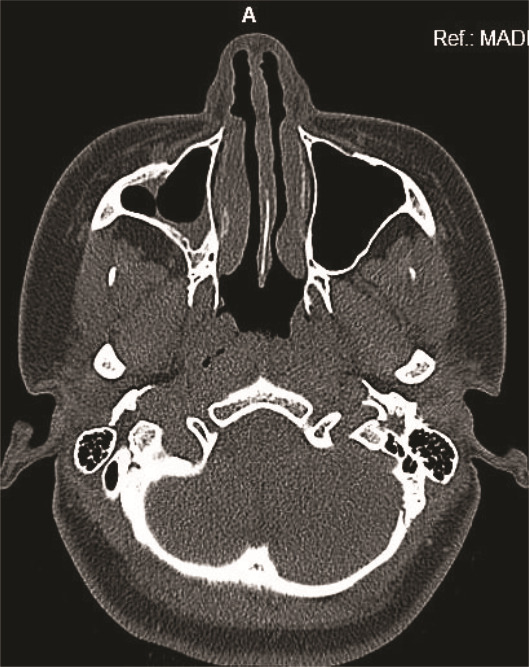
Postoperative computed tomography (CT) showing complete enucleation of the cyst and cystic contents
